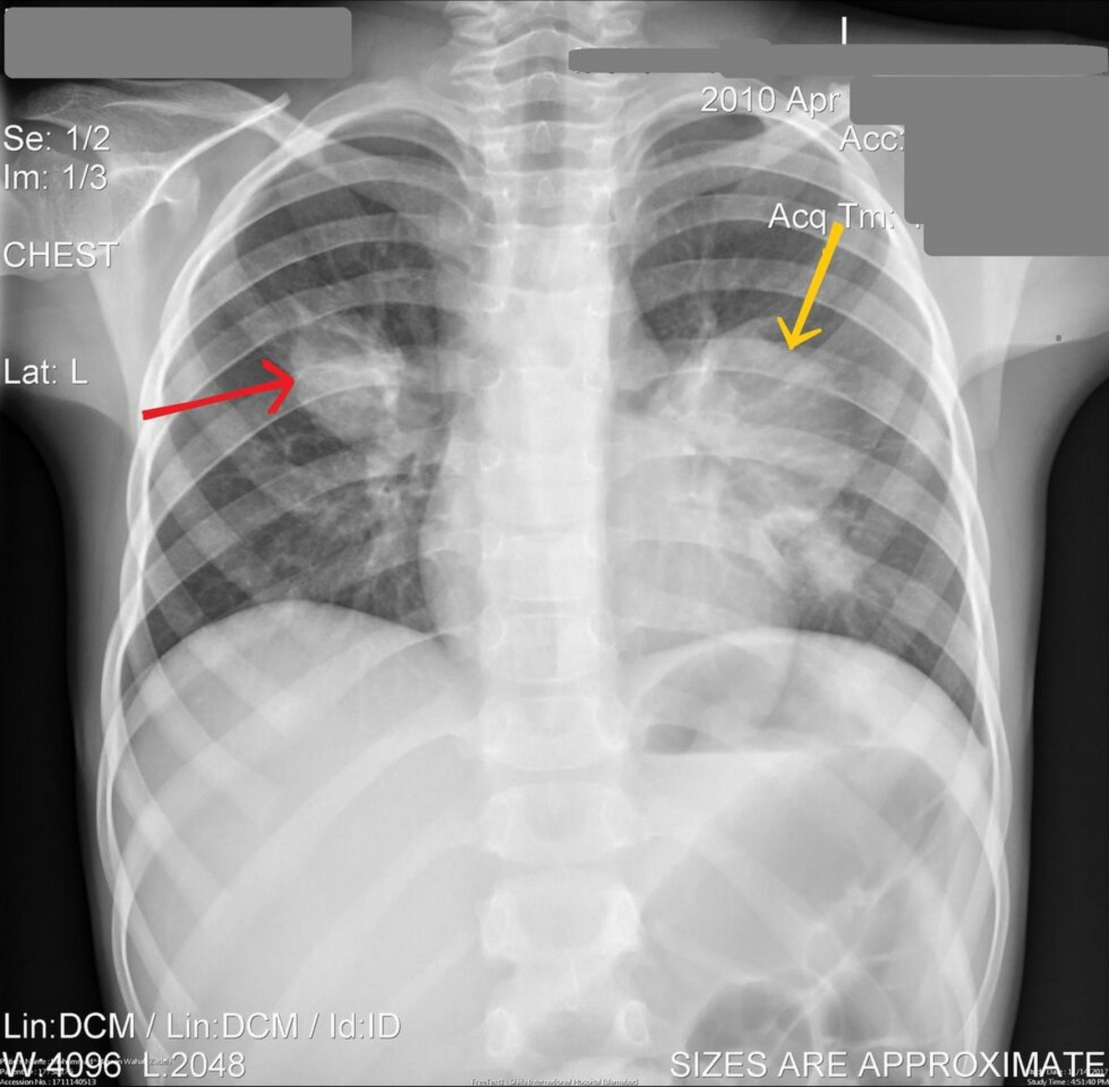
Chest X-ray anteroposterior (AP) view Arrows showing lobulated soft tissue densities in both lungs.
